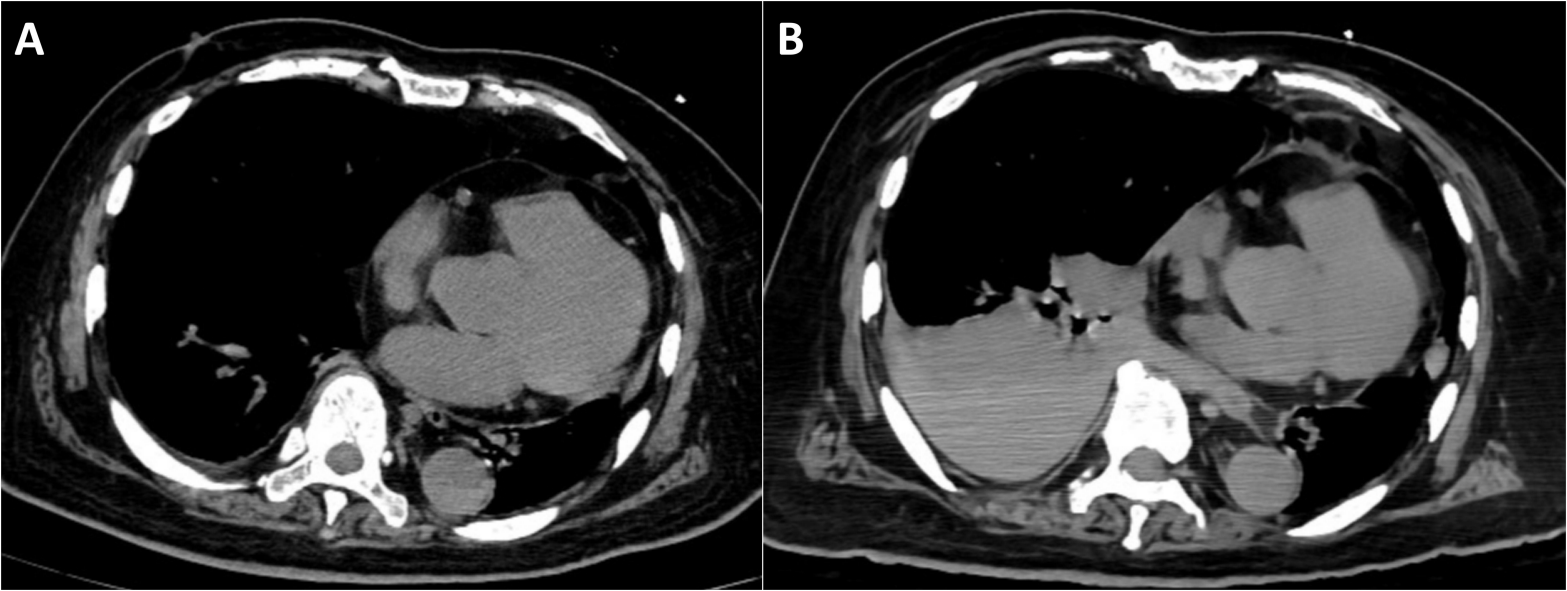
Chest computed tomography. (A) CT on the day of admission shows no significant pleural effusion. (B) Right-sided pleural effusion (possible hemothorax).
